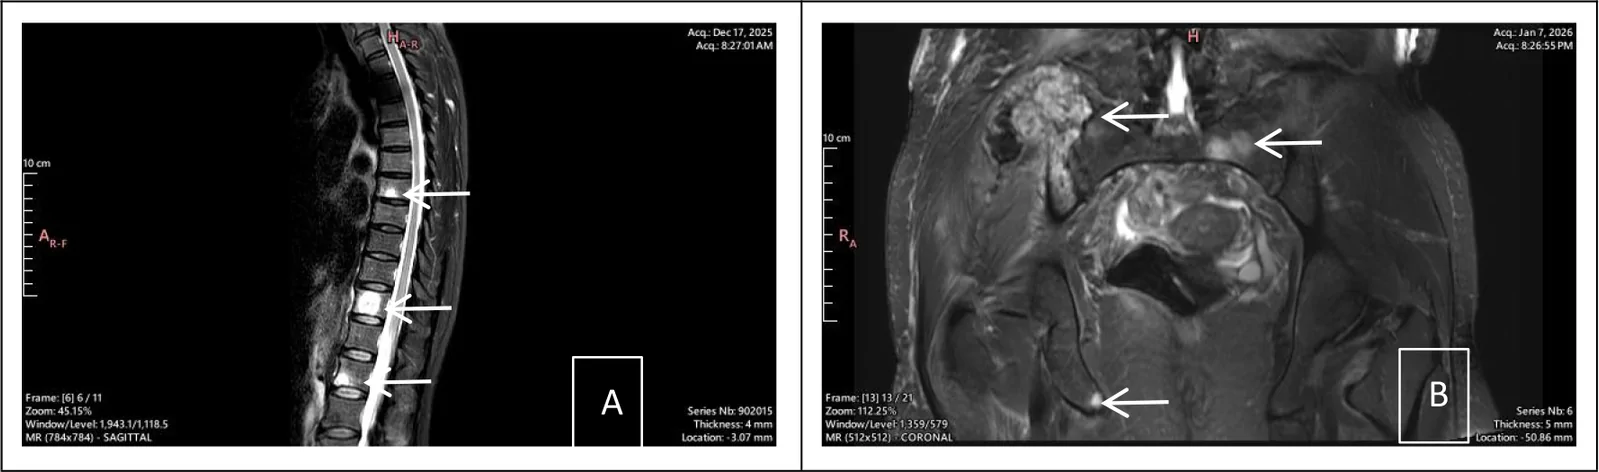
MRI findings. (A) Sagittal STIR of the thoracic spine, (B) Coronal STIR of the pelvis, showing multiple abnormal signal foci in multiple vertebral bodies and various bones of the pelvis, with a relatively large lesion in the right ilium and a surrounding soft tissue mass (as indicated by arrows).
